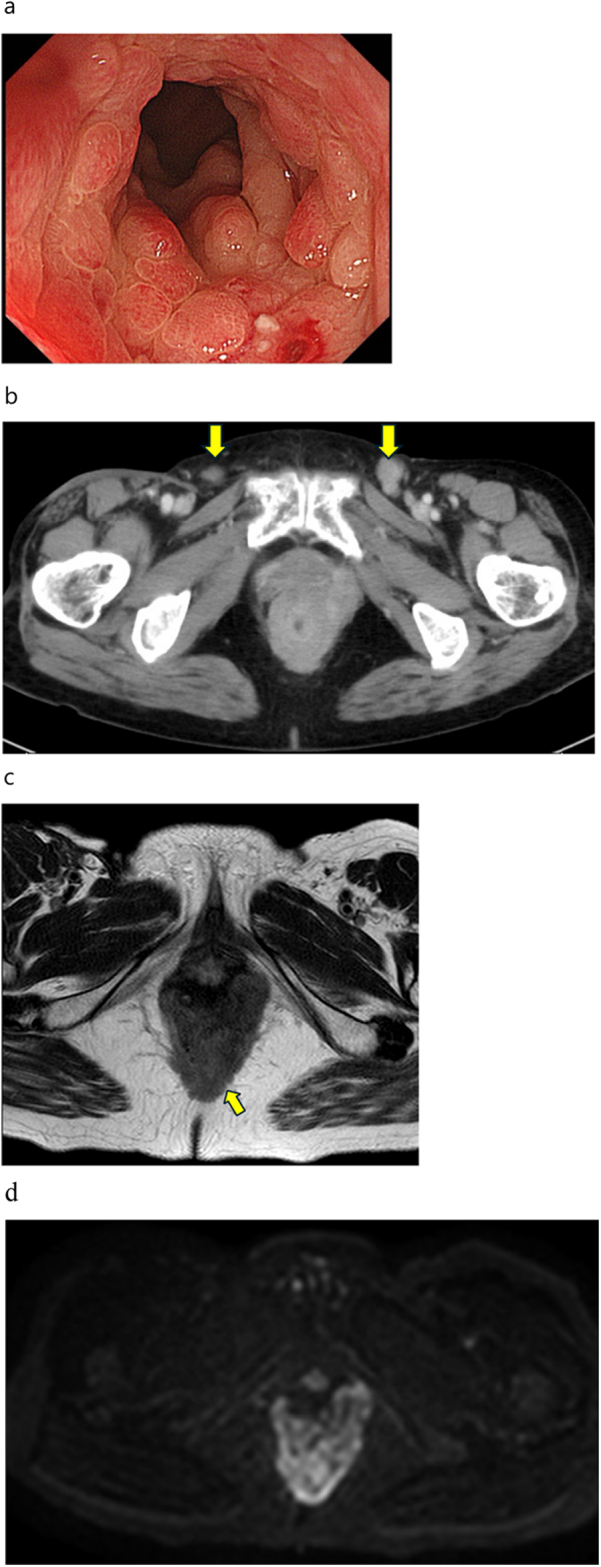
Endoscopic, computed tomography (CT), and magnetic resonance imaging (MRI) findings at diagnosis. a Fully circumscribed, indistinct border, type IV tumor in the anal canal. b Fully circumscribed wall thickening at the anal canal with metastasis to the bilateral inguinal lymph nodes (arrow). c Fully circumscribed wall thickening at the anal canal involving the anal sphincter in T2-weighted image (arrow). d The same slice as in c in diffusion-weighted image
